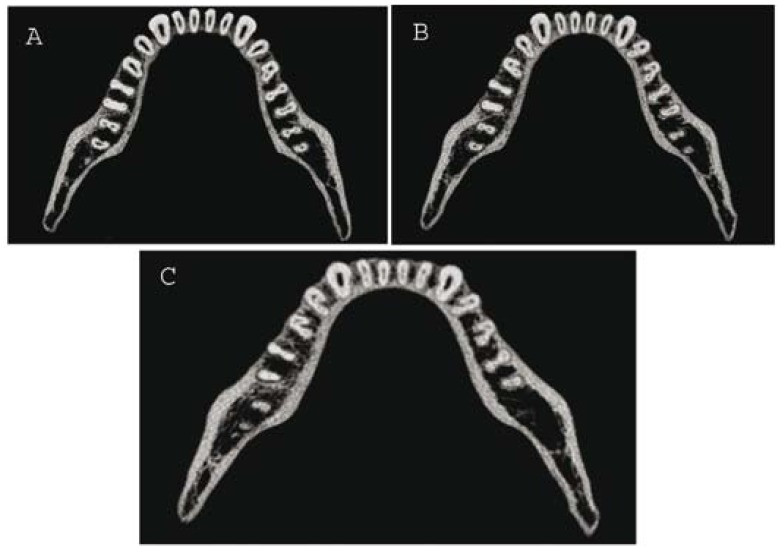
Axial CT slices; A) Coronal third section of the roots of mandibular premolars; B) Middle third section of the roots of mandibular premolars; C) Apical third section of the roots of mandibular premolars.
